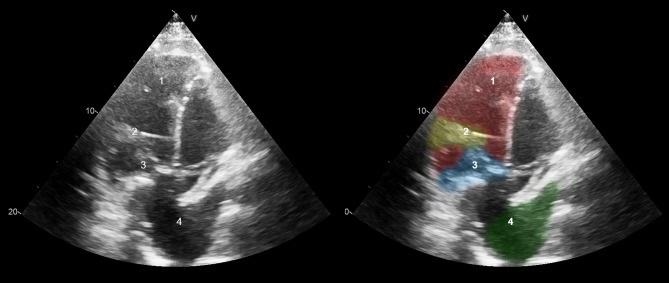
Transthoracic echocardiography of patient 1 after VAD implantation 1 systemic right ventricle 2 inflow cannula 3 tricuspid valve prosthesis 4 pulmonary venous tunnel
